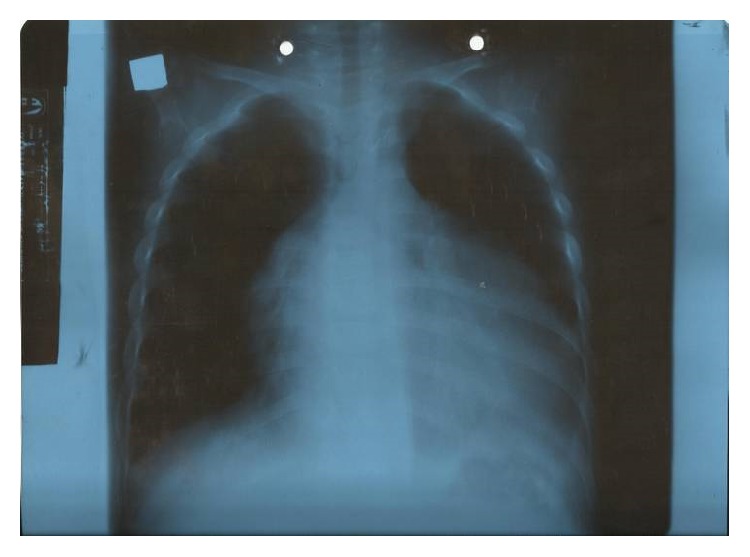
Plain film shows a “boot shaped” heart with an upturned cardiac apex due to right ventricular hypertrophy and concave pulmonary arterial segment.
